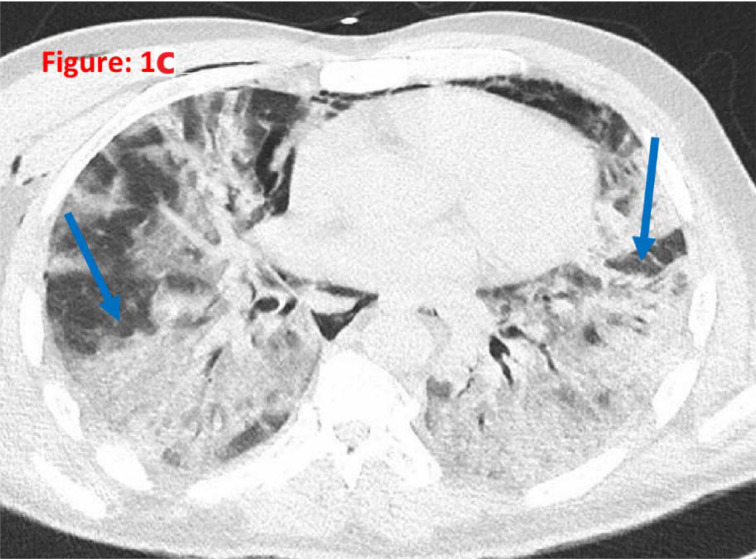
CT chest showing extensive fibrosis of the lung parenchyma and subpleural fibrosis, findings coinciding with COVID-19-associated interstitial lung disease.
